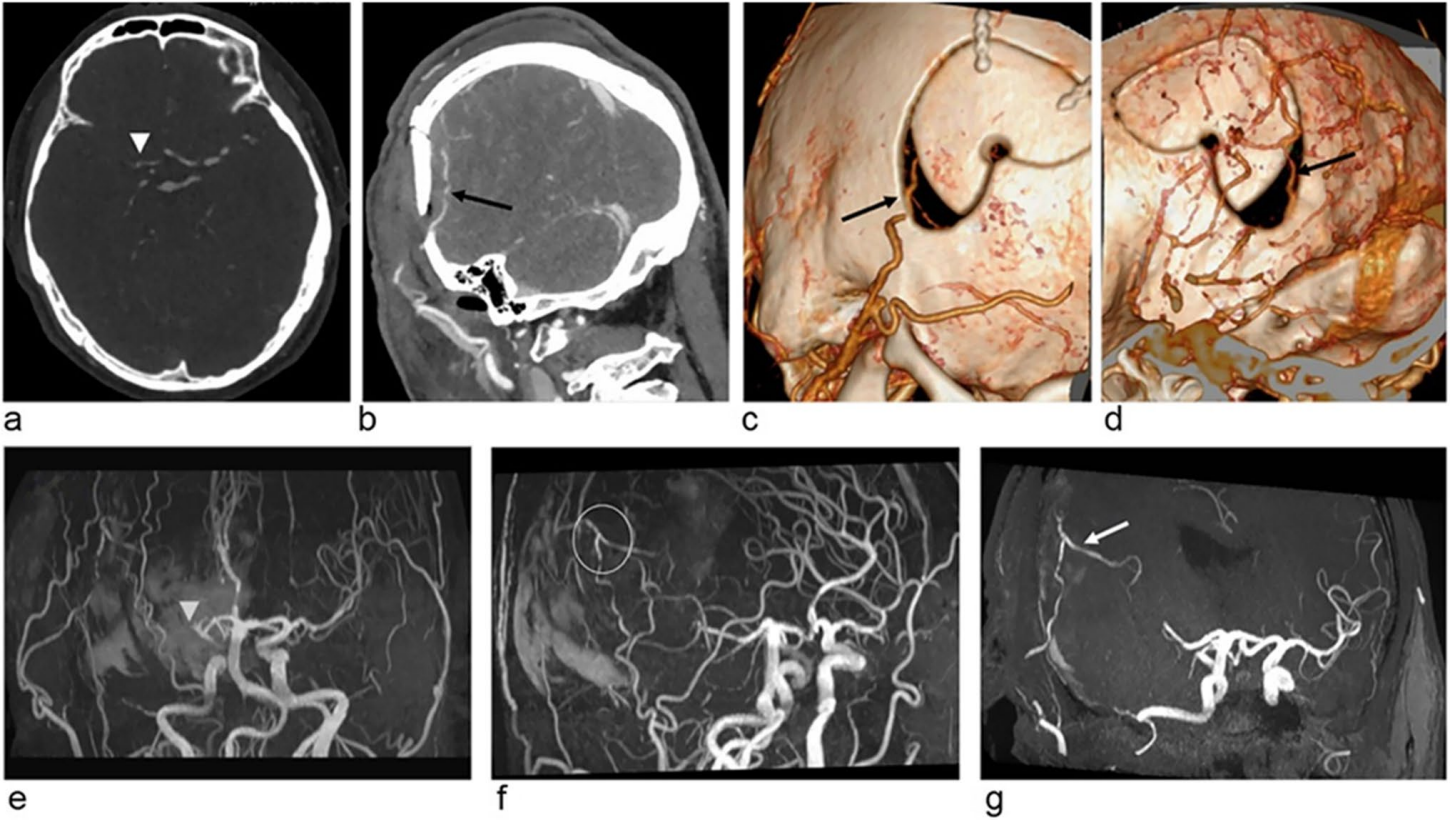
A 55-year-old patient underwent right-side STA-MCA bypass surgery. a Preoperative CTA and e preoperative MRA show the occlusion of the right MCA (arrowheads). b, c, d Postoperative CTA show that the right STA (black arrows) entered the cranium through the bone defect area of the temporal region. f and g Postoperative MRA clearly showed the anastomosis (circled area) and the distal branch of the MCA (white arrow). Image courtesy of Dr. Hanyu Jiang, Department of Radiology, West China Hospital
